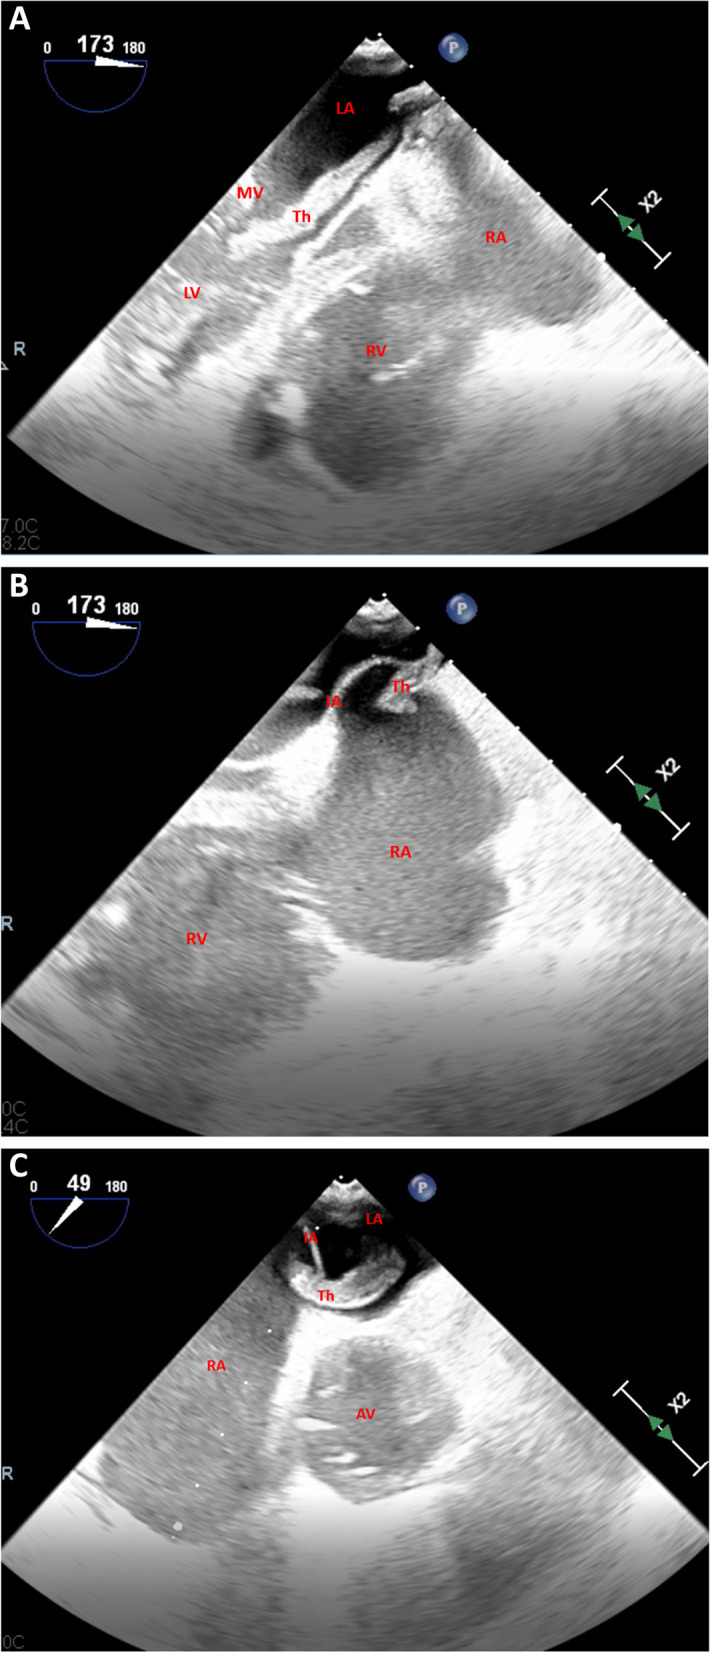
A-C Transesophageal echocardiogram; inverted four chamber midesophageal scan (A-B) shows dilated right chambers and a thrombus involving left atrium, right atrium and mitral valve; short axis midesophageal scan (C) shows thrombus crossing the interatrial septum. AV = aortic valve. IA = interatrial septum. LA = left atrium. MV = mitral valve. RA = right atrium. Th = thrombus
